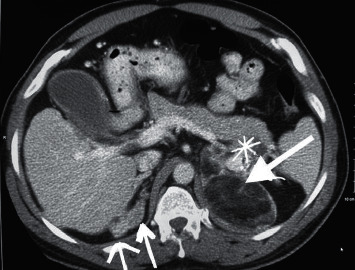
A contrast-enhanced computed tomography (CT) image demonstrating two nodules in the right adrenal gland (small arrows) and a large, left adrenal mass with calcifications (large arrow) and suspected invasion of the left adrenal mass into the tail of the pancreas (asterisk).
